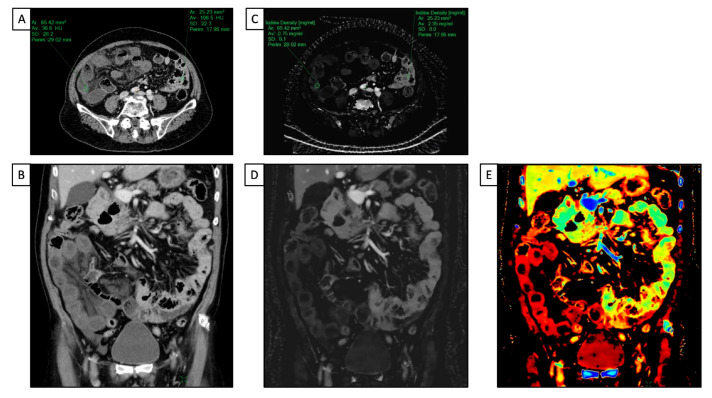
A 66 y-o male with diffuse abdominal pain underwent abdominal CT with a final diagnosis of distal ileum bowel ischemia. (A,C) Axial and coronal conventional CT images acquired after intravenous contrast media injection show a slight difference in attenuation value (HU) of the small bowel walls, with reduced enhancement in the distal ileum and regular enhancement in jejunum and proximal ileum; (B,D) iodine maps clearly show the difference in iodine uptake between the normal walls of the jejunum and proximal ileum and the poor iodine uptake of the distal tract of the ileum; (E) the Z-effective map allows us to better and more simply define the different enhancement in terms of colors between healthy and ischemic bowels due to the atomic number of iodine.
